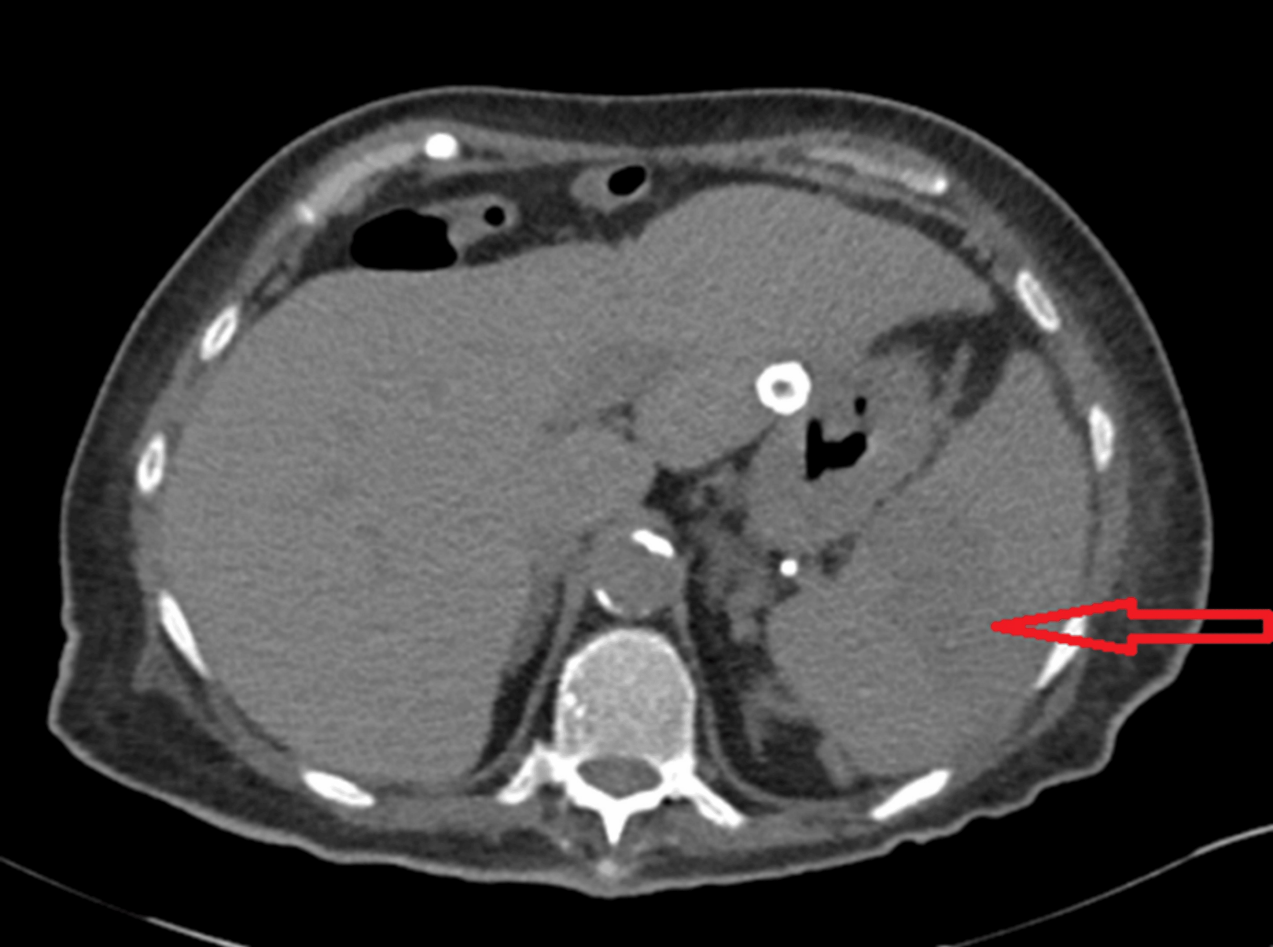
CT abdomen showing splenic infarction in a patient with chronic myeloid leukemia (CML) Axial Computed Tomography image of the abdomen demonstrating a linear area of hypoattenuation in the central spleen (arrow), consistent with splenic infarction. This radiologic finding confirmed the suspected diagnosis and prompted initiation of systemic anticoagulation after risk-benefit assessment. The spleen appears enlarged, a common finding in chronic myeloid leukemia due to extramedullary hematopoiesis and leukemic infiltration.
